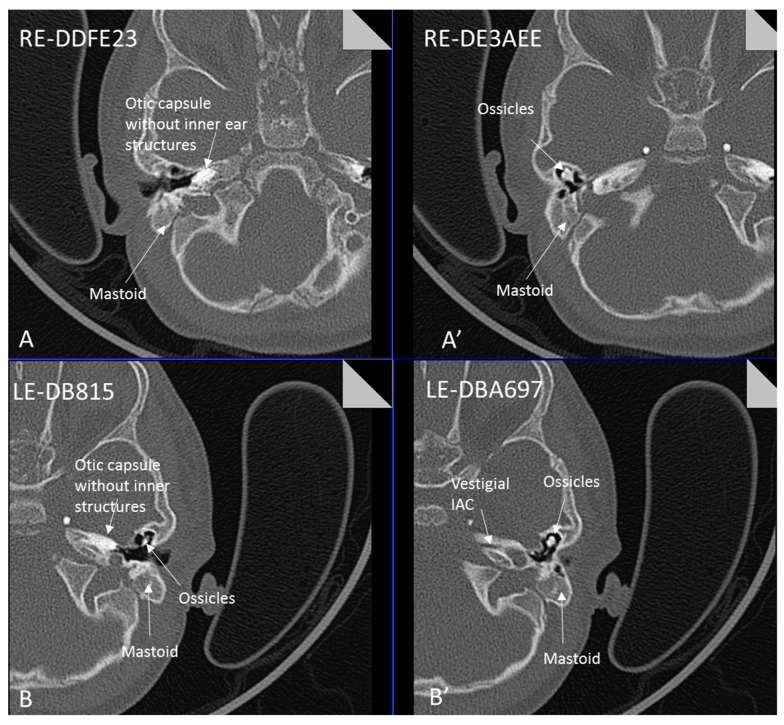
Axial CT petrous bone in patient 8 of the right (A,A′) and left (B,B′) ears shows complete labyrinthine aplasia (Michel deformity) characterised by absence of the inner ear structures: cochlea, vestibule, semicircular canals and vestibular and cochlear aqueducts. The otic capsule is hypoplastic and only a vestigial IAC is observed in the left ear (B′). Middle ear ossicles are bilaterally present and normal.
